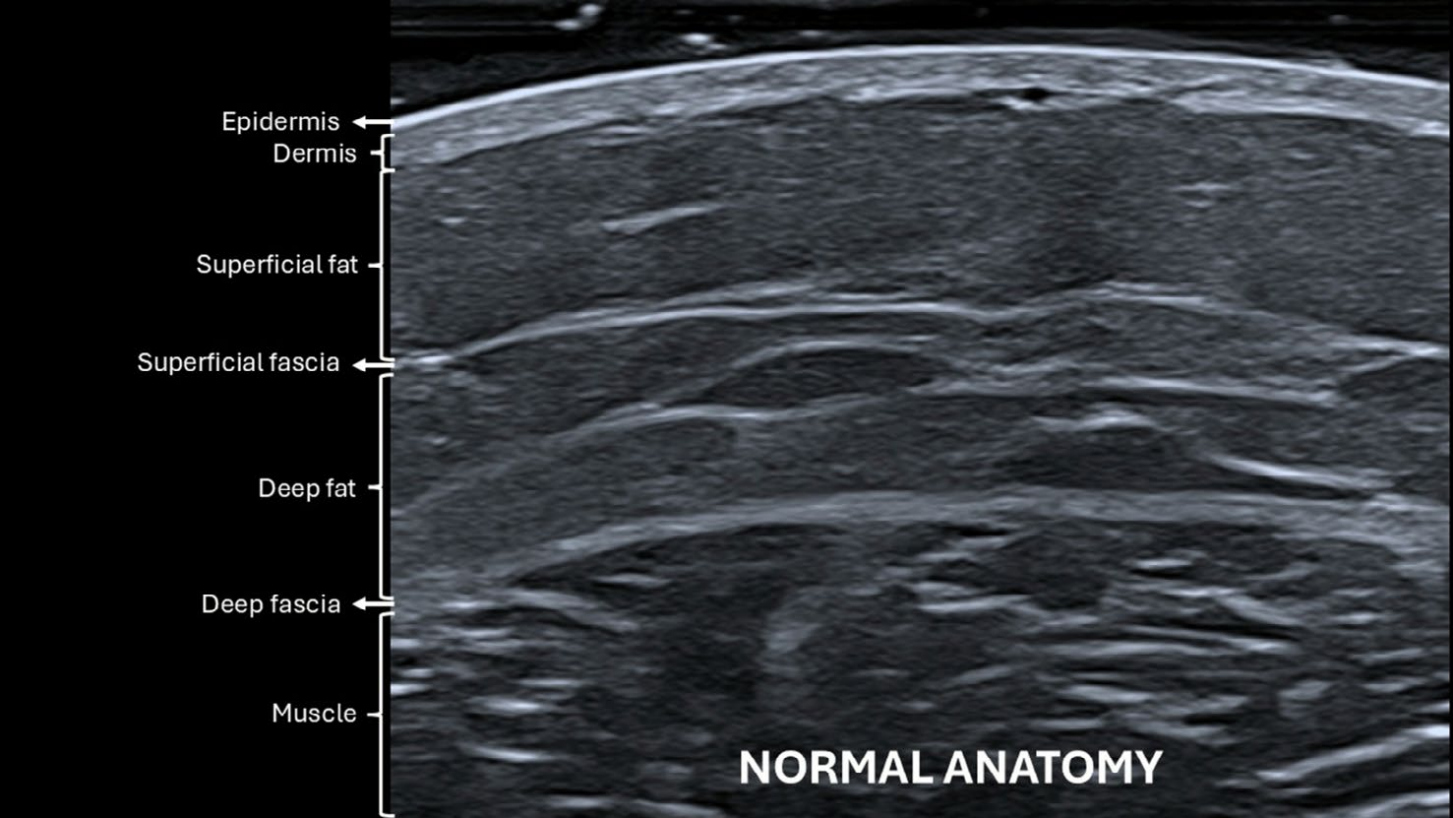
B‐mode ultrasound image (20 MHz) showing normal gluteal anatomy prior to treatment.
